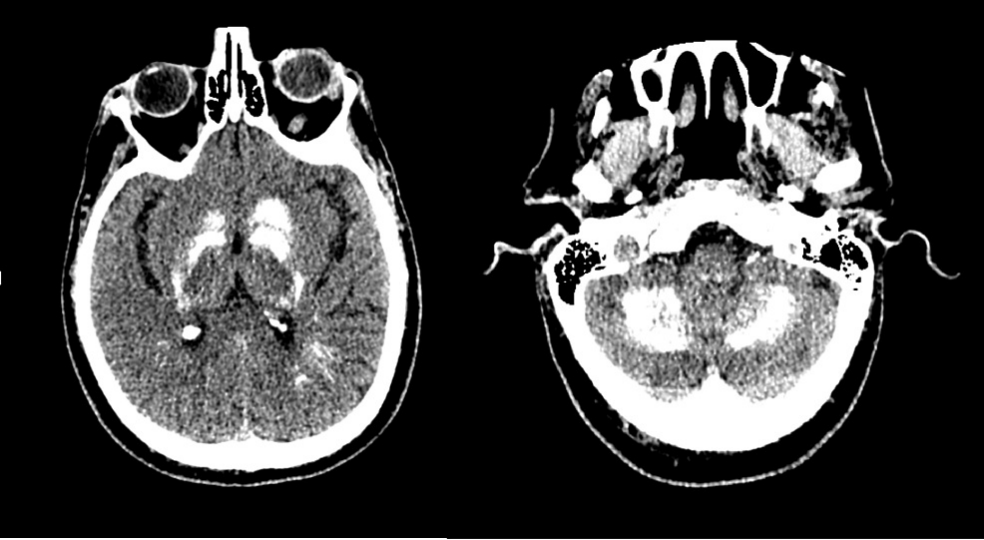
Cranial CT demonstrating important calcification in the basal ganglia and cerebellum.
